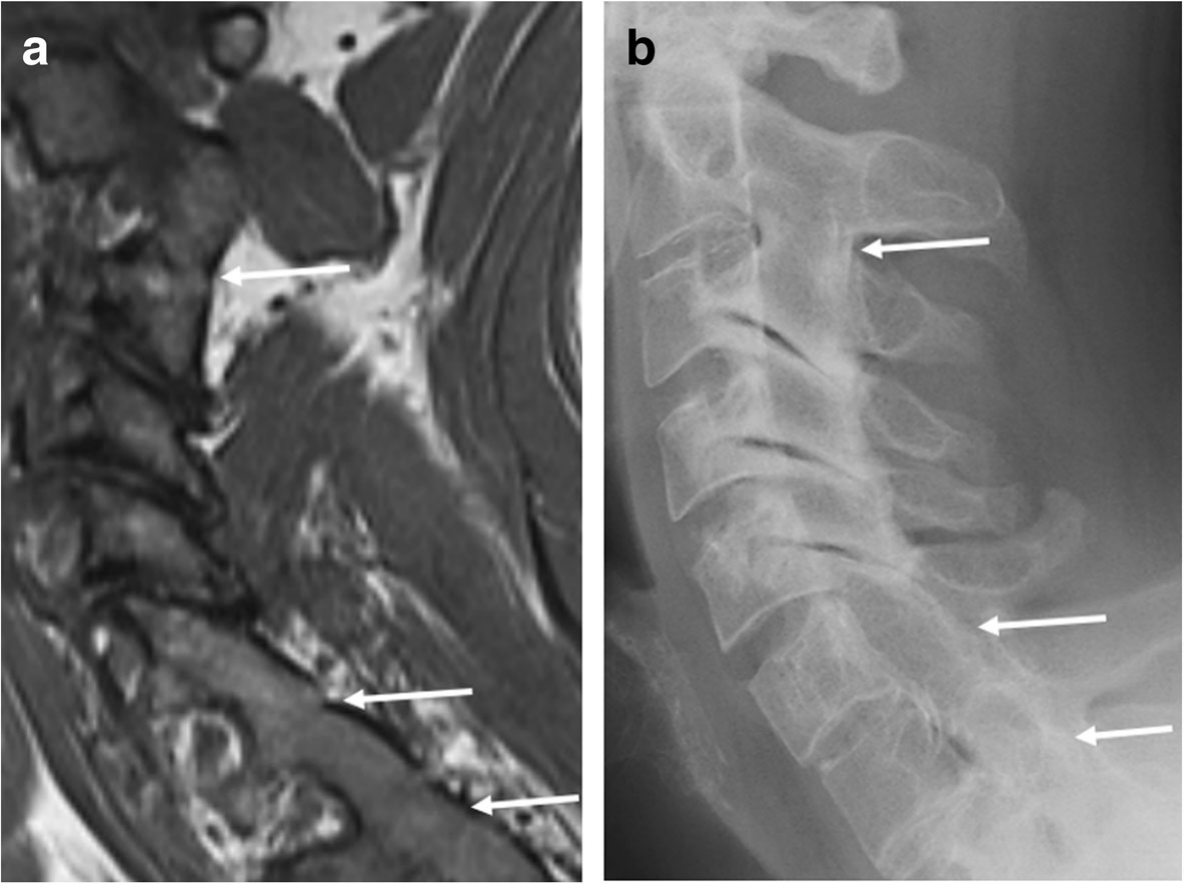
Ankylosis of the facet joints. a Sagittal T1-weighted MR image acquired in a 34-year-old man with SpA shows ankylosis of the facet joints of C2-C3, C6-C7, and C7-Th1 (arrows). b Radiography of the cervical spine confirms this bony fusion of these joints (arrows)
